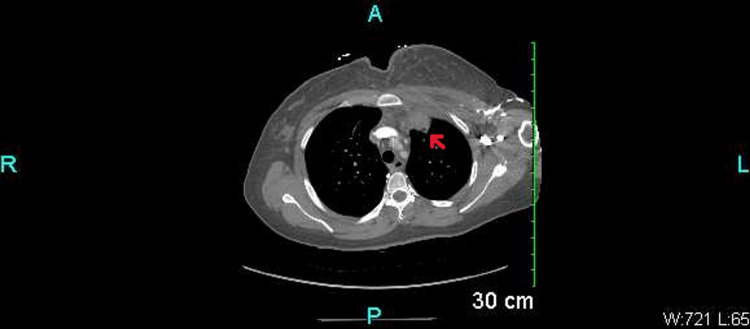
CT chest 3 weeks later The image shows a 33 mm x 33 mm new periosteal reaction and destructive bone loss.
